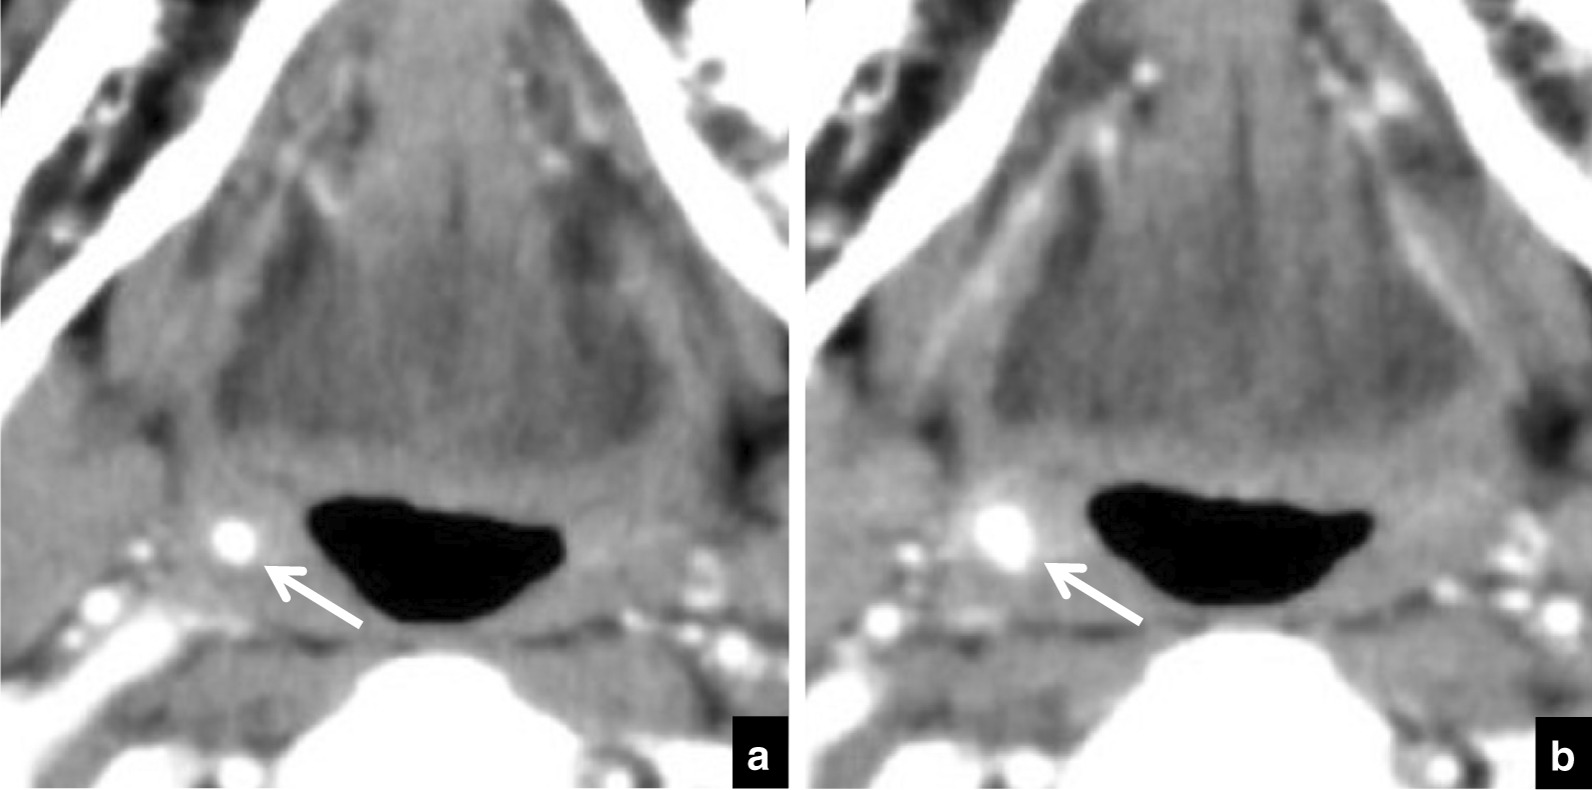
A 70-year-old subject with malignant tumor of the tongue in whom tonsillolith size increased on CT. a Axial CT images at the palatine tonsillar level at the initial examination. The arrow indicates a tonsillolith. The long axis of the tonsillolith was 1.4 mm. b Follow-up CT image at the same level 42 months later. The long axis of the tonsillolith (arrow) has increased to 2.4 mm
